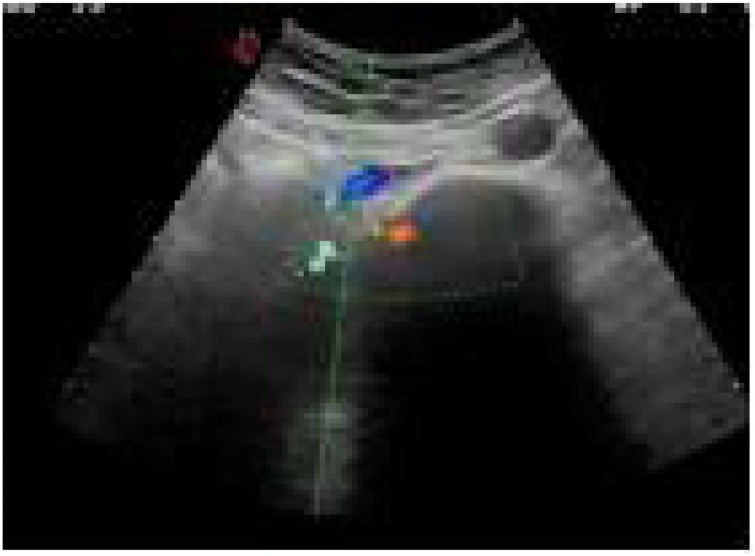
(A): Doppler ultrasound showing complete resolution of the thrombus.
